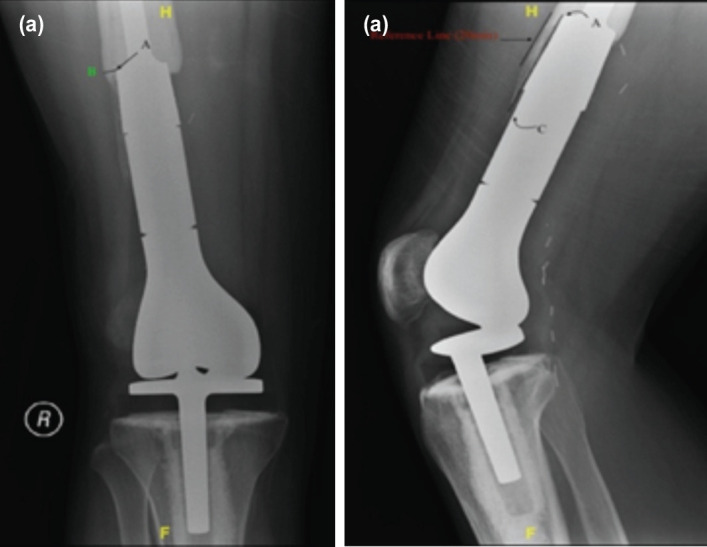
Measurement of thickness and length of extracortical bone bridge interface. (a) Antero-posterior radiograph of Knee joint shows measurement of EBBI thickness (Point A to Point B). (b) Lateral radiograph of Knee joint shows measurement of EBBI Length (Point A to Point C).
